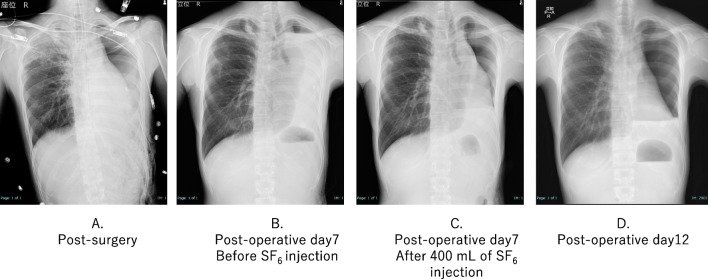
A X-ray showed that the mediastinal shift was corrected immediately after the surgery. B Air and pleural fluid in the left thoracic cavity were absorbed, leading to progressive mediastinal shift to the left by the seventh postoperative day. C 400 mL of SF6 gas was carefully injected into the left thoracic cavity while monitoring the patient's symptoms. D Five days after the initial SF6 injection, as the gas expanded according to plan, the volume of the left thoracic cavity increased, resulting in further correction of the mediastinal shift
